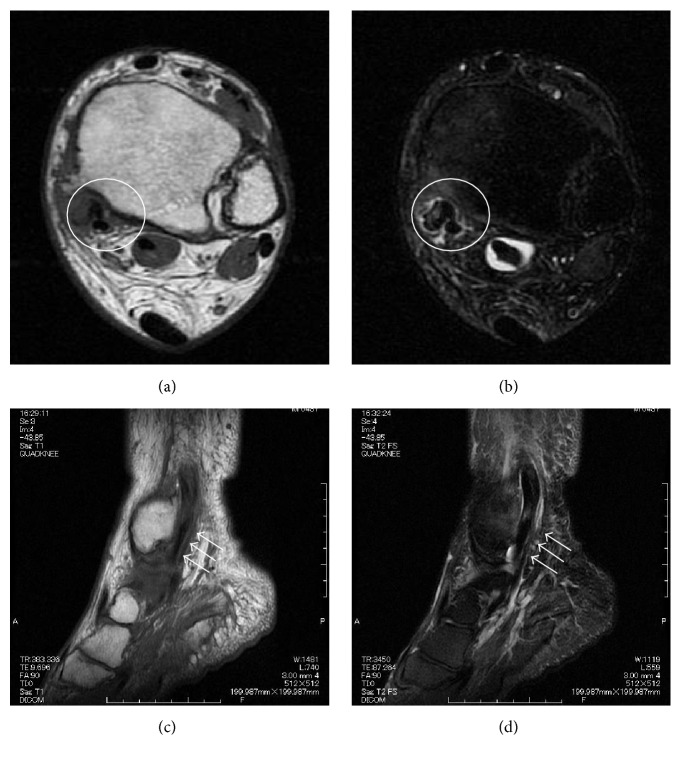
Magnetic resonance imaging (MRI). (a) T1- and (b) T2-weighted represent axial images. (c) T1- and (d) T2-weighted represent sagittal images. Circle and arrow show the posterior tibial tendon.
